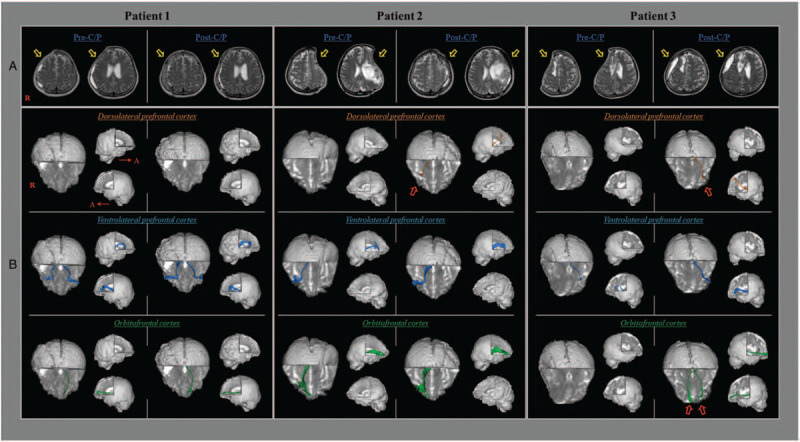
(A) T2-weighted brain magnetic resonance images of three women with hemorrhagic stroke before (pre-C/P) and after cranioplasty (post-C/P) (yellow arrows: OP side). (B) Diffusion tensor tractography (DTT) of the dorsolateral prefrontal cortex, the ventrolateral prefrontal cortex, and the orbitofrontal cortex of the prefronto-thalamic tract. The non-reconstructed dorsolateral prefrontal cortices of patients 2 and 3 on the contra-OP side and the orbitofrontal cortex of patient 3 on both sides on pre-C/P DTT were able to be reconstructed on post-C/P DTT (red arrows).
